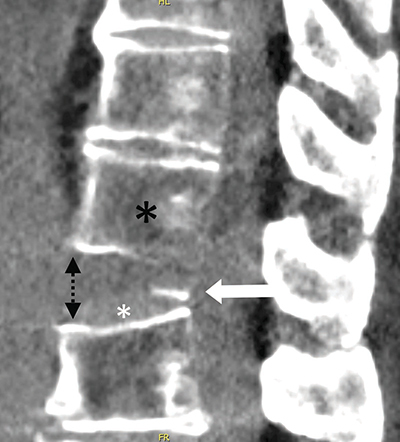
Sagittal CT-scan image showing a partial avulsion of the inferior endplate (arrow) of T12 (black star), which has remained attached to L1, along with the slightly hyperdense disc (white star). There is a diastasis between the anterior parts of the vertebral bodies (black dotted arrows) compared to the posterior part.
